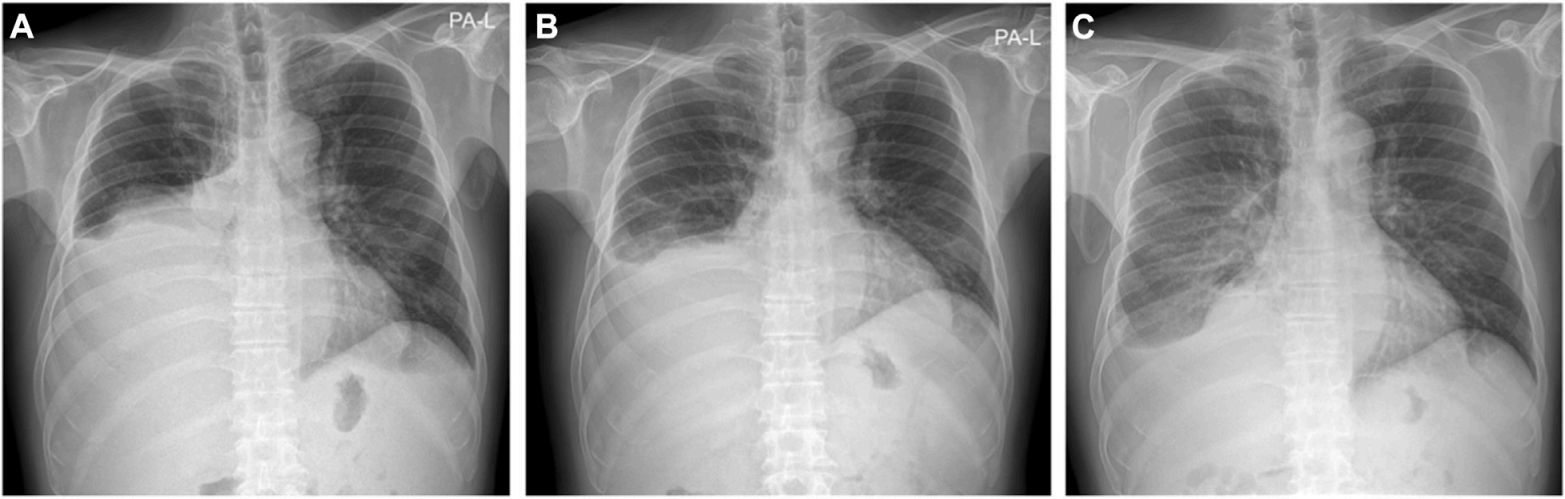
Chest X-ray images. (A) Chest X-ray on July 10 (B) Chest X-ray on October 23 (C) Chest X-ray on December 10. Even after he stopped taking diuretic from July 27, the chest X-ray findings above show decrease of pleural effusion.
